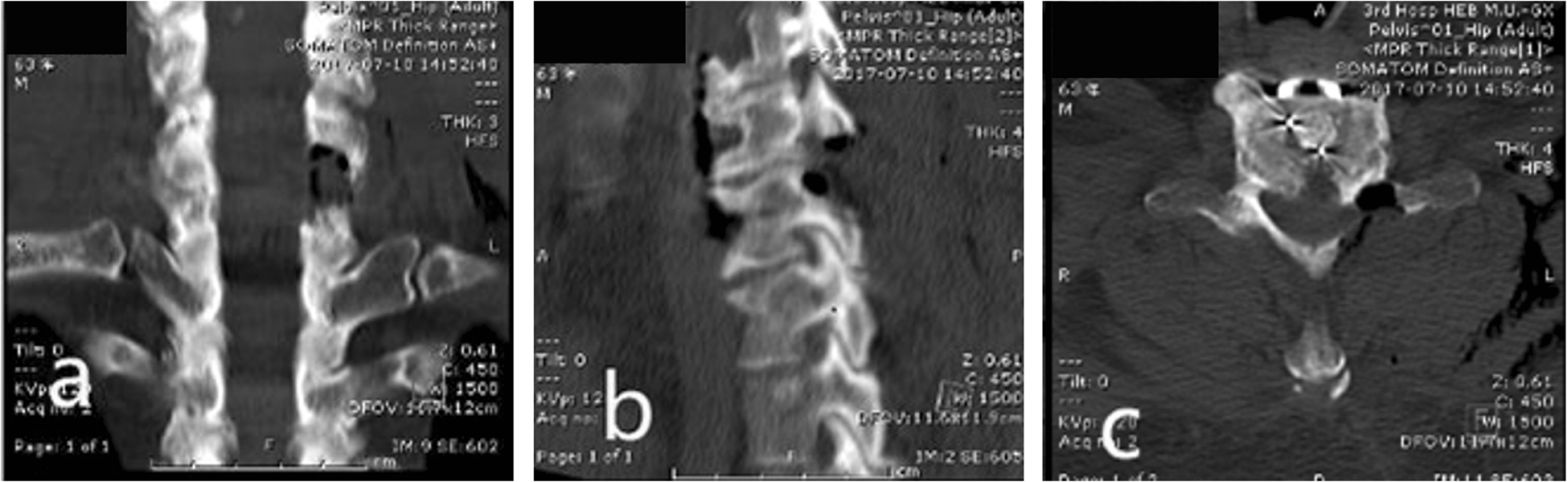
The CT imaging changes after cervical6–7 Key-hole surgery. a coronal, b sagittal, c transverse
